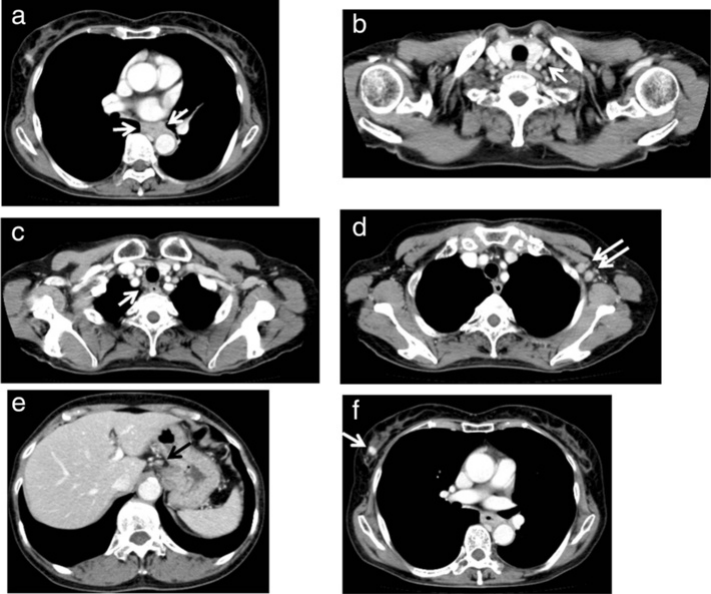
Chest and abdominal computed tomography at admission. a Wall thickening in the middle thoracic esophagus. b The left supraclavicular lymph node (7.0 mm in the short-axis plane). c The right recurrent nerve lymph node (7.0 mm in the short-axis plane). d Lymph node metastases in the left axillary space (13.8 and 14.7 mm in the short-axis plane). e Lymph node along the lesser curvature of the stomach (6.7 mm in the short-axis plane). f Mass of the right breast (maximum diameter of 16 mm)
